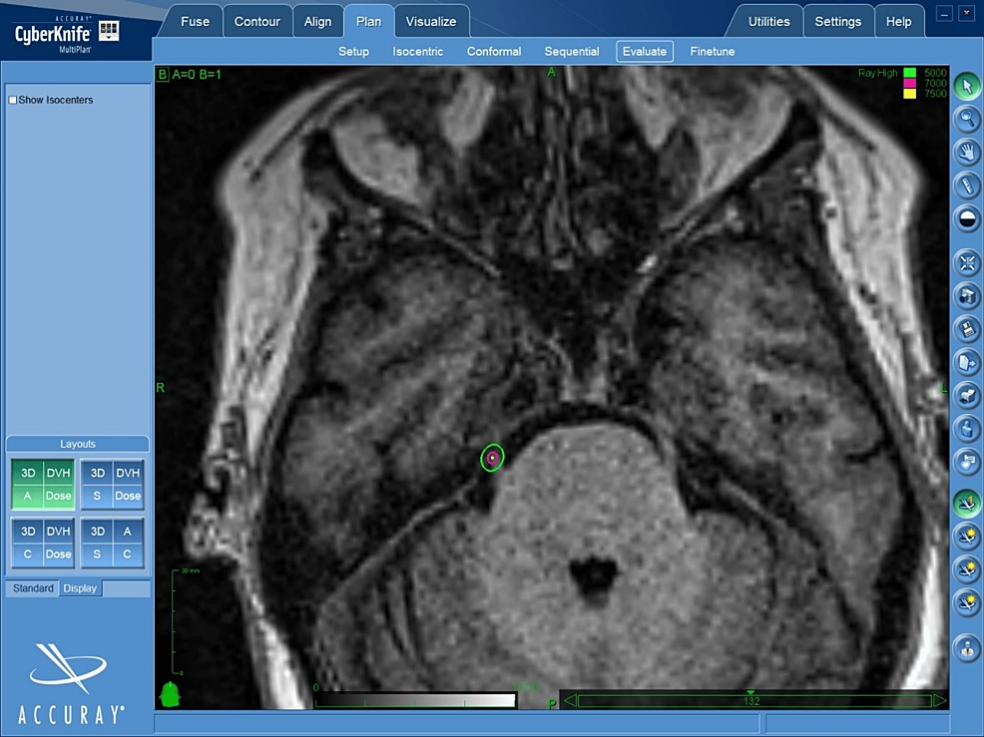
T1 MRI showing the trigeminal nerve at the level of the cisternal segment of the posterior fossa and the radiation dose prescribed for the case of a 45-year-old patient with a history of right trigeminal neuralgia in its three branches (V1, V2 and V3), previously treated with GK at a dose of 90Gy at 100%. The patient is identified as number 8 in Table 2, and has also been described previously.
